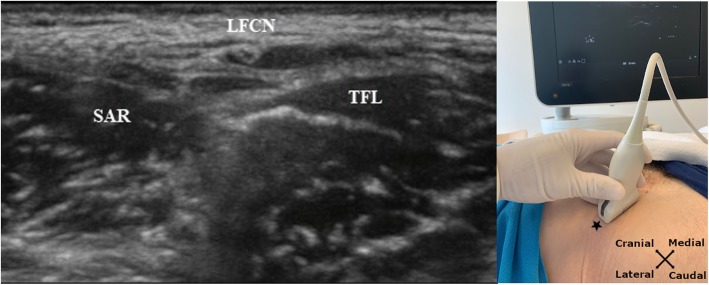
Ultrasound image of the target nerve. The image is not from an actual trial participant. SAR = sartorius muscle. LFCN = lateral femoral cutaneous nerve. TFL = tensor facia latae muscle. * marks the anterior superior iliac spine
